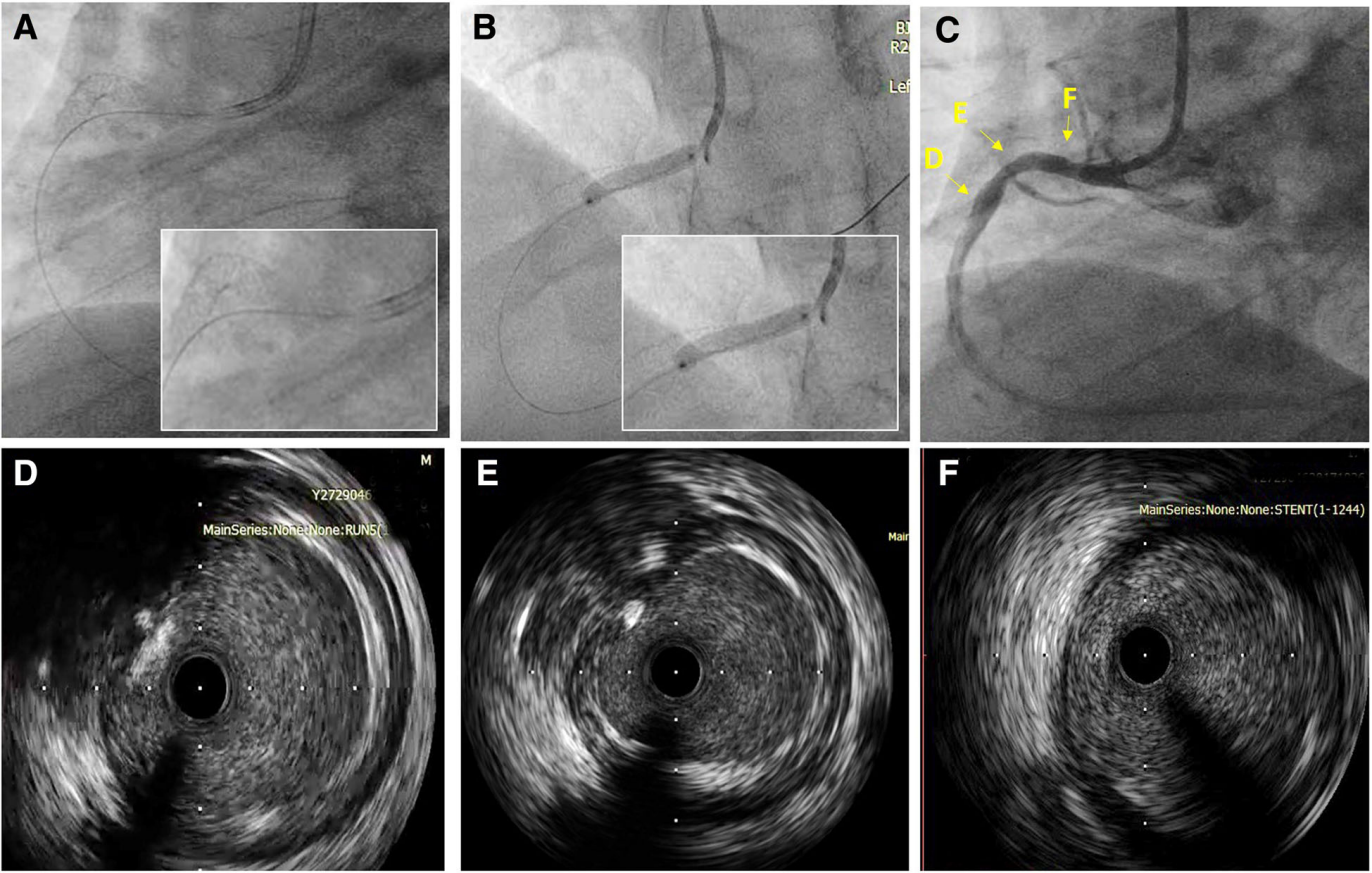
Coronary angiography and intravascular ultrasound. a Wire re-entry into true lumen of right coronary artery. b Coronary angiography revealed that the wire was in true lumen of right coronary artery. c Coronary angiography after true lumen stent implementation. d–f Intravascular ultrasound confirmed that wire was in the true lumen from distal, mid, and proximal right coronary artery respectively
